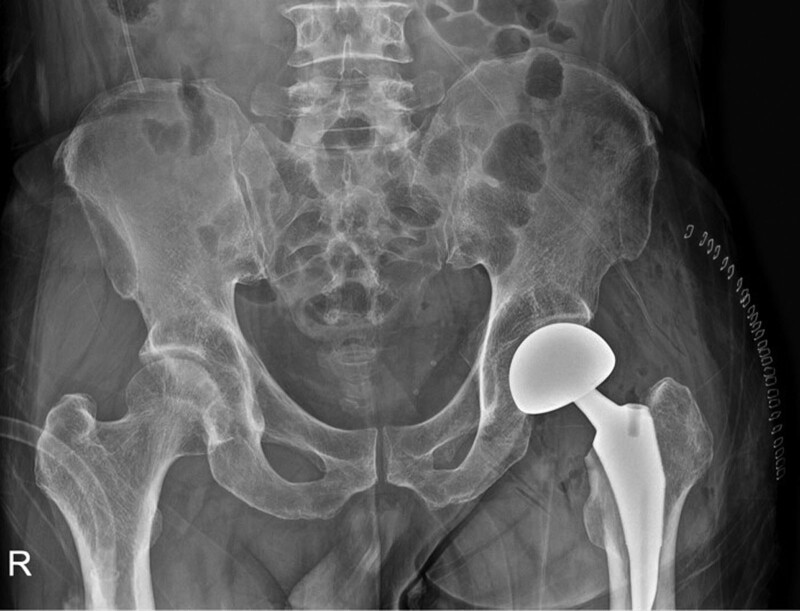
Patient initial postoperative anteroposterior radiograph of hip hemiarthroplasty.
